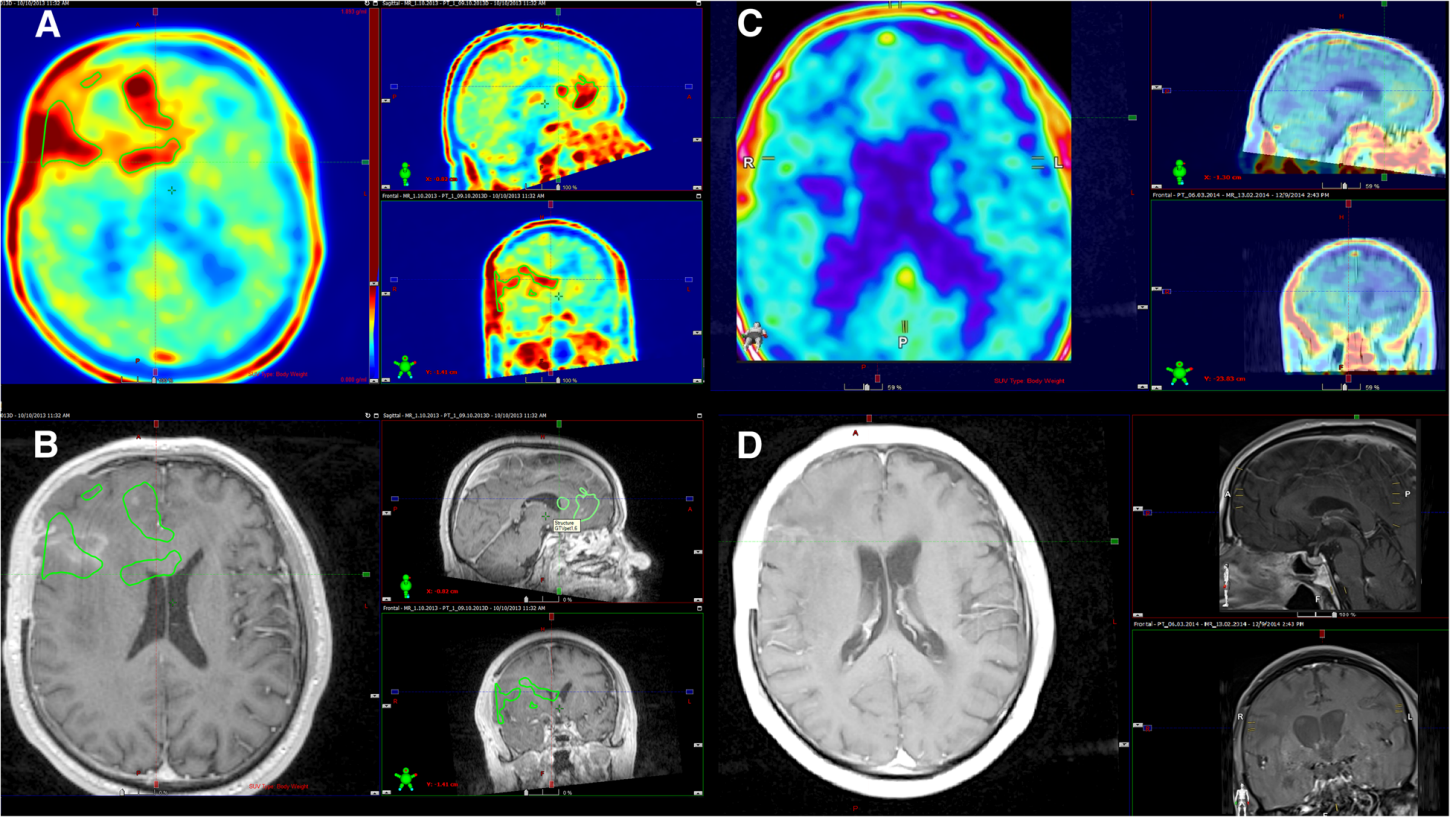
Comparison of pre- and post-irradiation dtp FET PET and MRI images of glioblastoma long-term survival in the right frontal lobe. Dtp FET PET volume < 40 cm^3^. The large pathological uptake volume extends into the SVZ over a substantial area (a). Contrast-enhanced T1-weighted MRI performed for radiotherapy planning with no SVZ infiltration in the axial, sagittal, and coronal planes (b). The patient had a favorable outcome, being alive at the end of the observation period (OS 47 months) without progression. Twelve months post-treatment dtp FET PET with complete response: TBR below 1.6 (c) and MRI with residual contrast enhancement (d)
